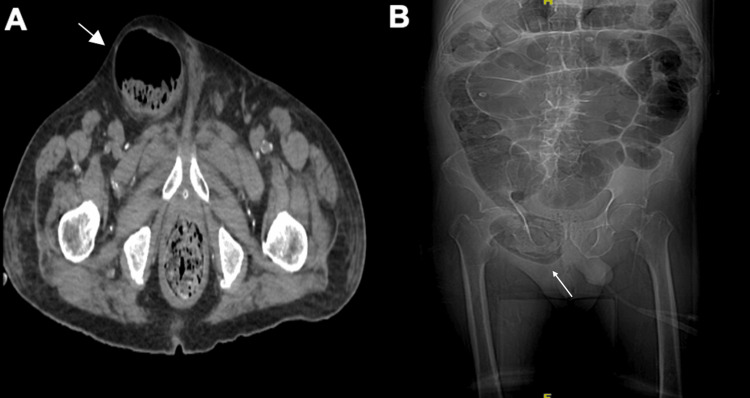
A. Axial abdominal computed tomography with Amyand's hernia (CT). B. Coronal CT scan demonstrating the hernial defect in the inguinal region.
